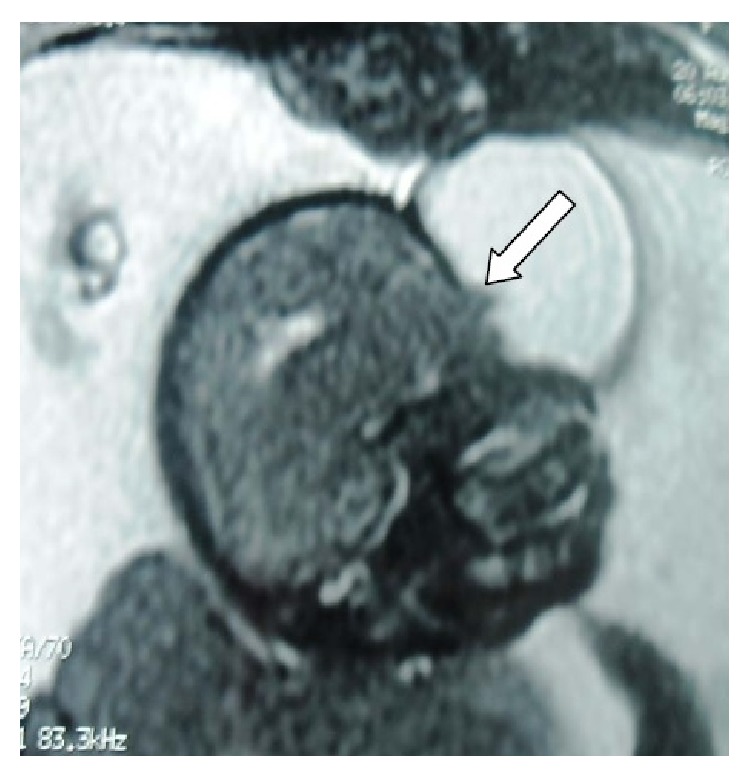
Prenatal MRI showing a well-defined osseous defect in the underlying calvarium (arrow).
